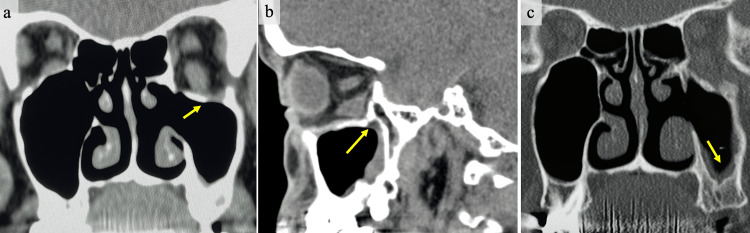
Postoperative CT findings. (a) A coronal CT scan shows that the orbital floor has been reconstructed, effectively containing the orbital contents within the orbit, as indicated by the yellow arrow. (b) A sagittal CT scan shows that the repair extends from the anterior to the posterior edge of the orbit, as indicated by the yellow arrow. (c) Odontogenic maxillary sinusitis improved following endoscopic sinus surgery and tooth extraction.
